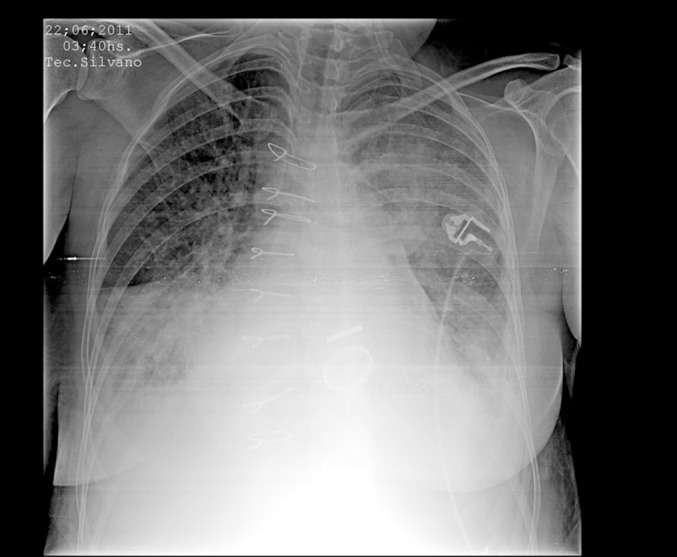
Chest X-ray revealing improvement of the radiological picture after
